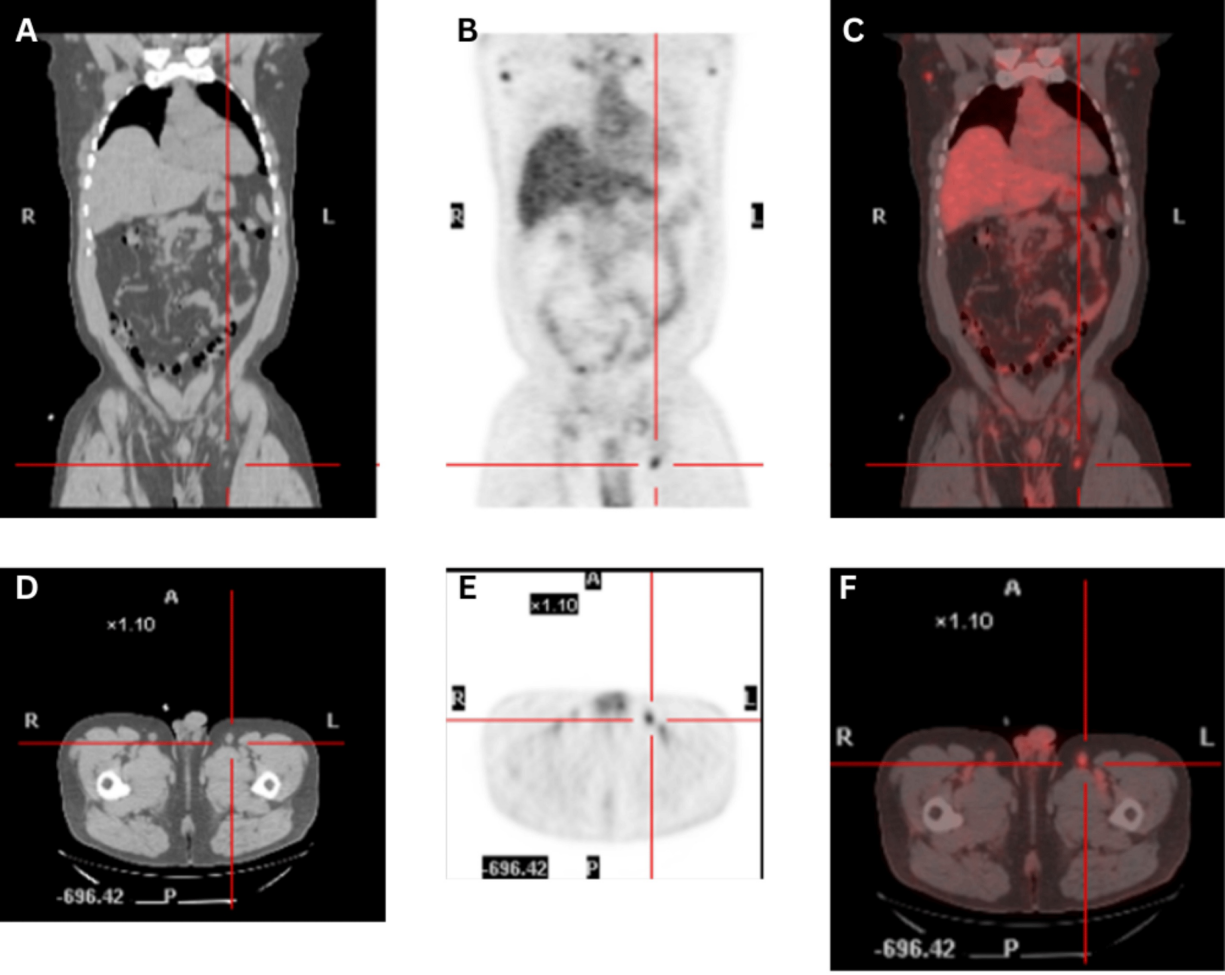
Intensely FDG-avid left inguinal lymph node on PET/CT (A) CT Coronal view. (B) PET Coronal view. (C) Fused Coronal view. (D) CT Transaxial view. (E) PET Transaxial view. (F) Fused Transaxial view.
